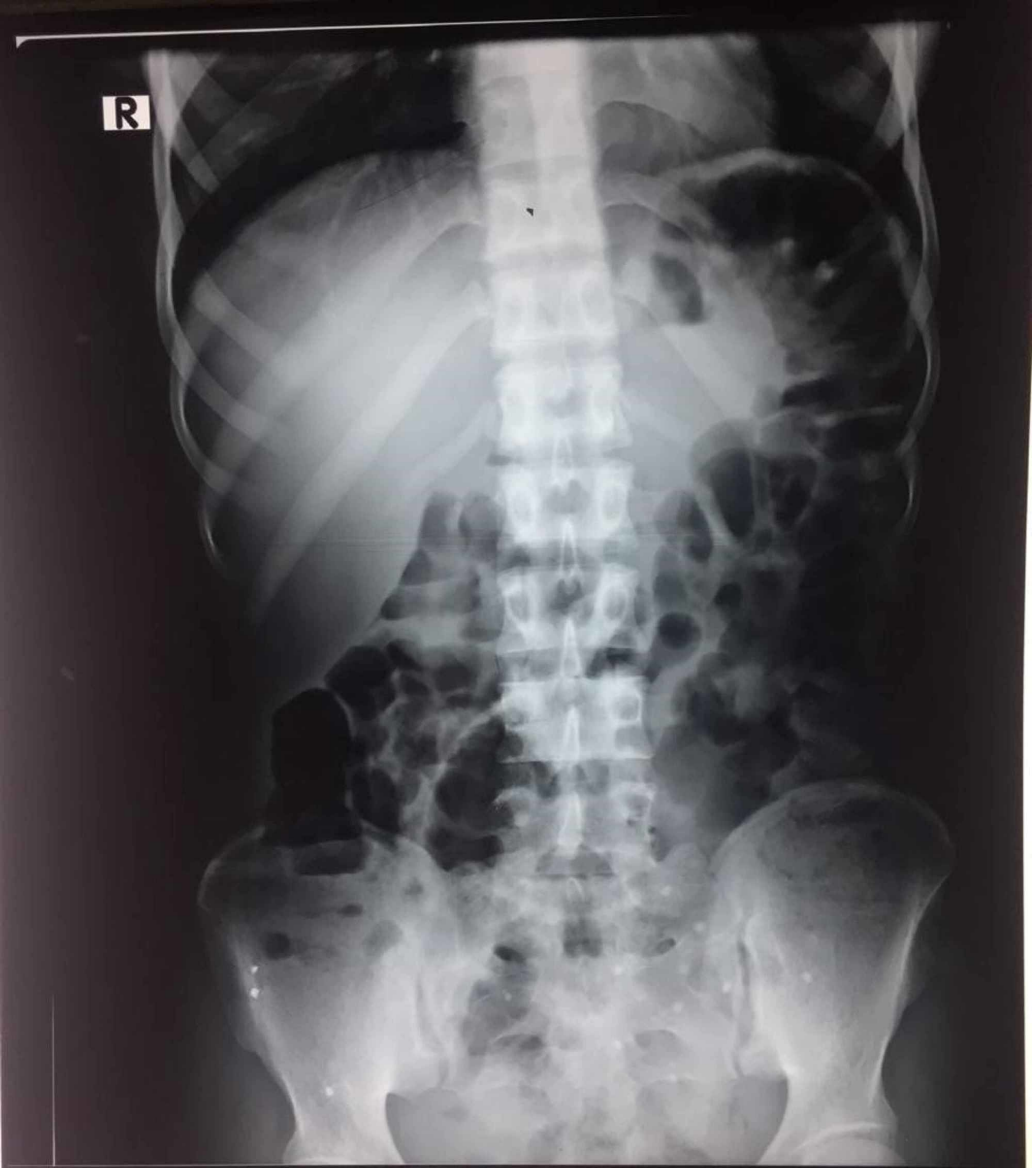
X-ray (abdomen and pelvis) depicting dilated small gut loops.
